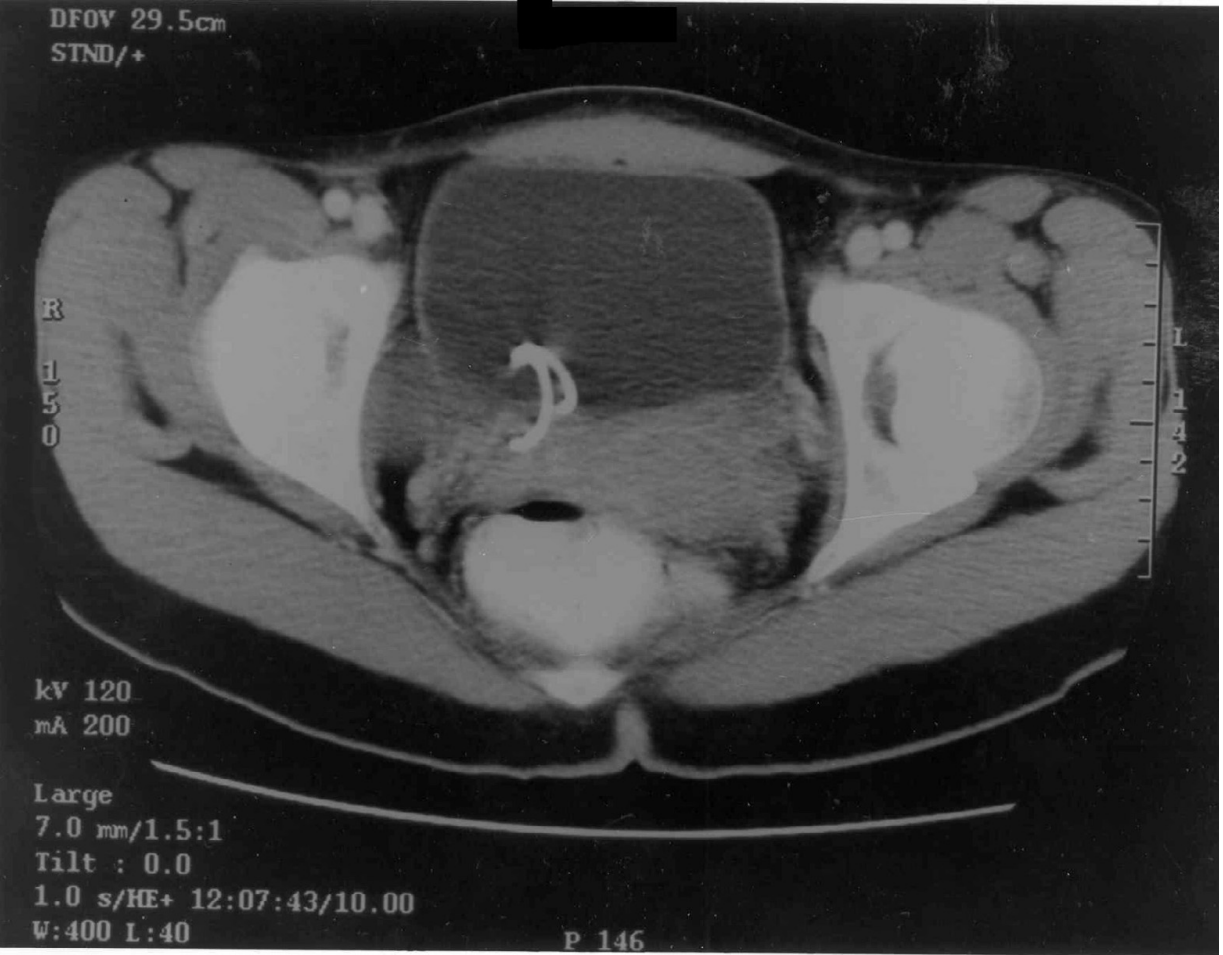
CT scan of the abdomen/pelvis at 4 months post op showing a normal-looking bladder with right double J stent in place.
